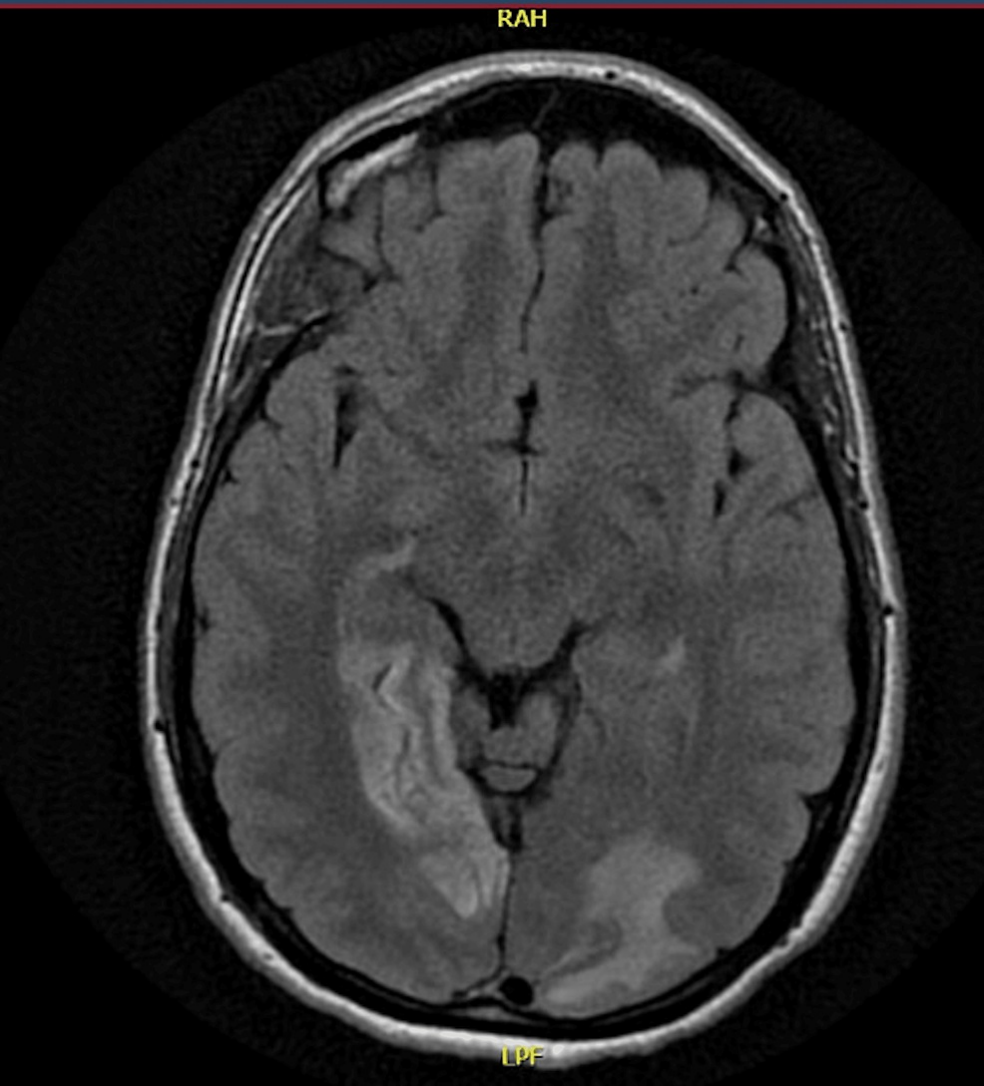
MRI done showing acute-subacute, mildly enhancing ischemia/infarction along the posterior medial right temporal and occipital lobe cortices
